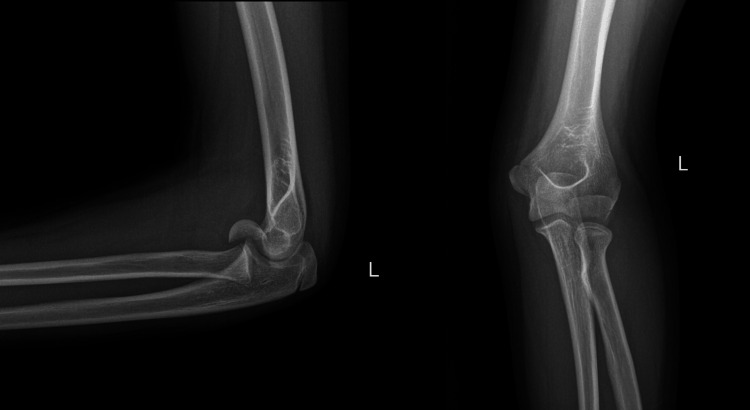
Preoperative anteroposterior and lateral radiograph depicting the capitellar fracture. The double arc sign is present. The olecranon and the medial epicondyle have not been fused yet.
